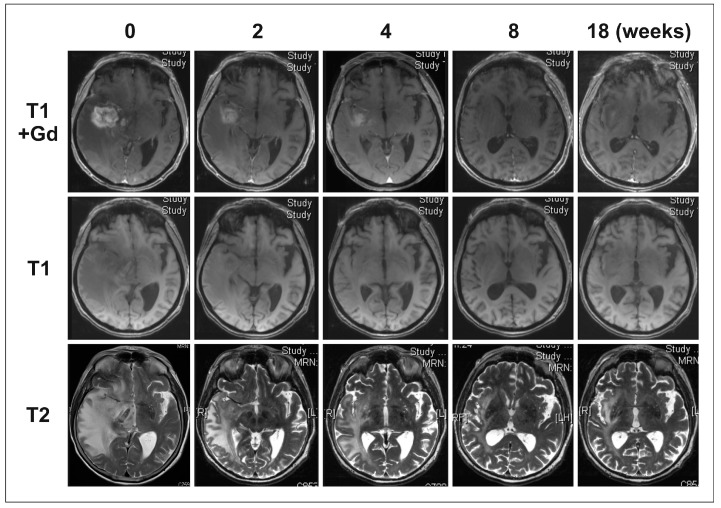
Exemplary magnetic resonance imaging (MRI) of Patient 7: contrast enhancing lesion. T1 sequences with and without Gadolinium contrast enhancer and T2 sequences were obtained at Weeks 0, 2, 4, 8 and 18 after BEV therapy initiation. After BEV therapy initiation contrast enhancing and T2 hyperintensive alterations decreased. At Week 18, a small contrast-enhancing alteration formed and the T2 hyperintensive alterations were increasing.
